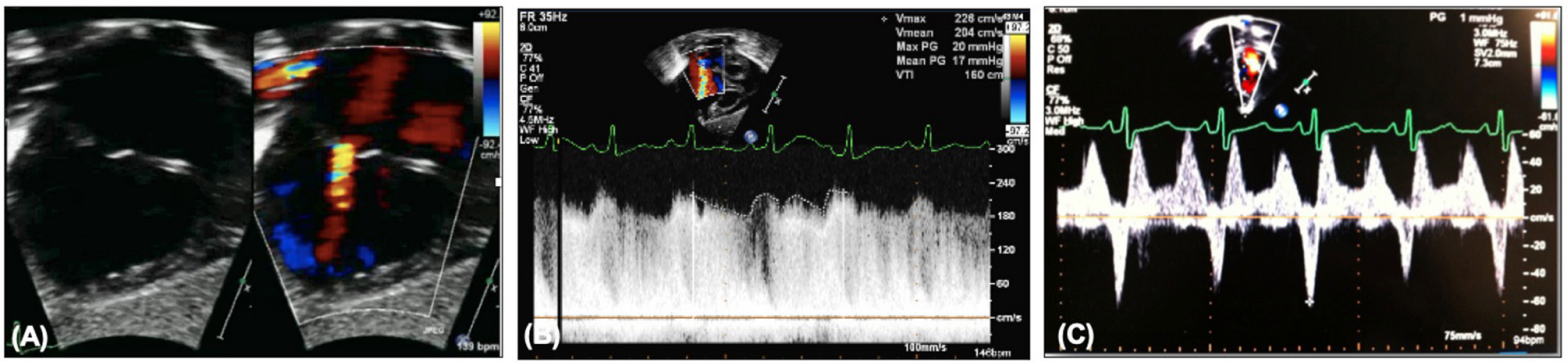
(A) Restrictive atrial communication on color. (B) Elevated transatrial Doppler mean gradient. (C) A wave reversal on pulmonary vein Doppler.
